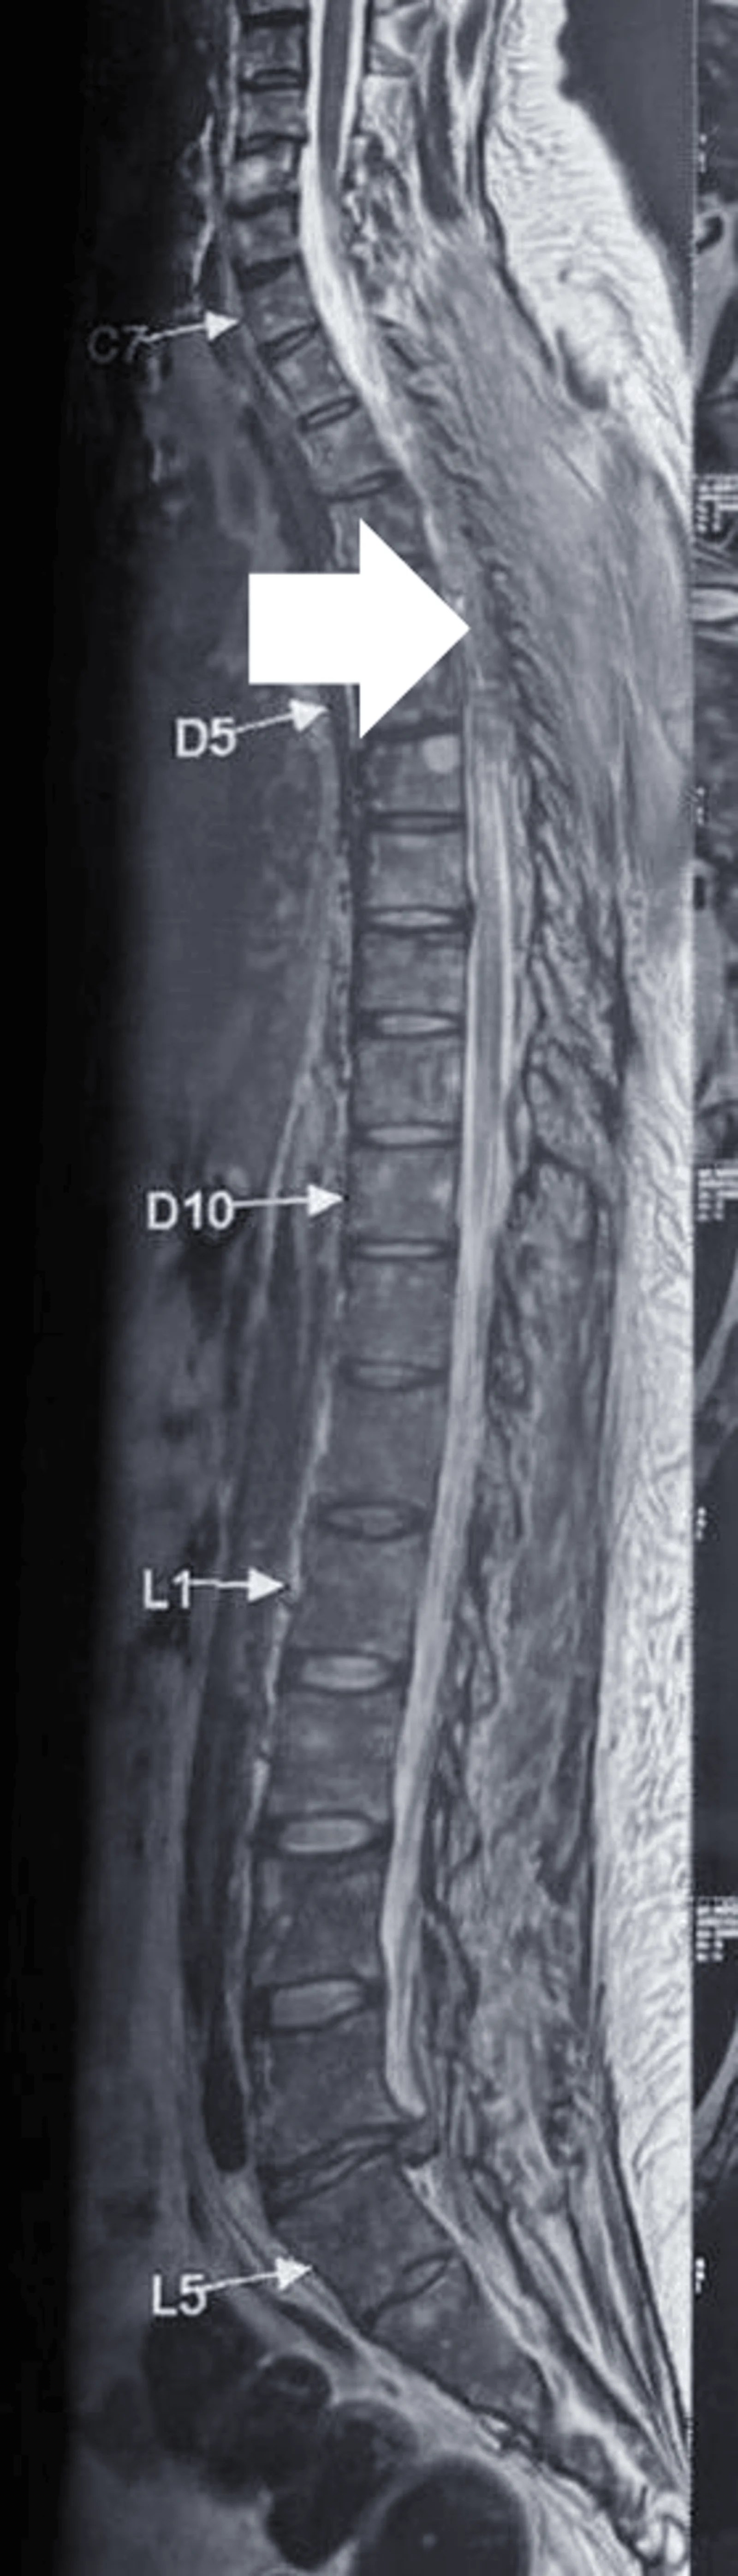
Magnetic resonance imaging of the spine showing an epidural abscess extending from C7 to D6. Arrow indicating epidural abscess.
